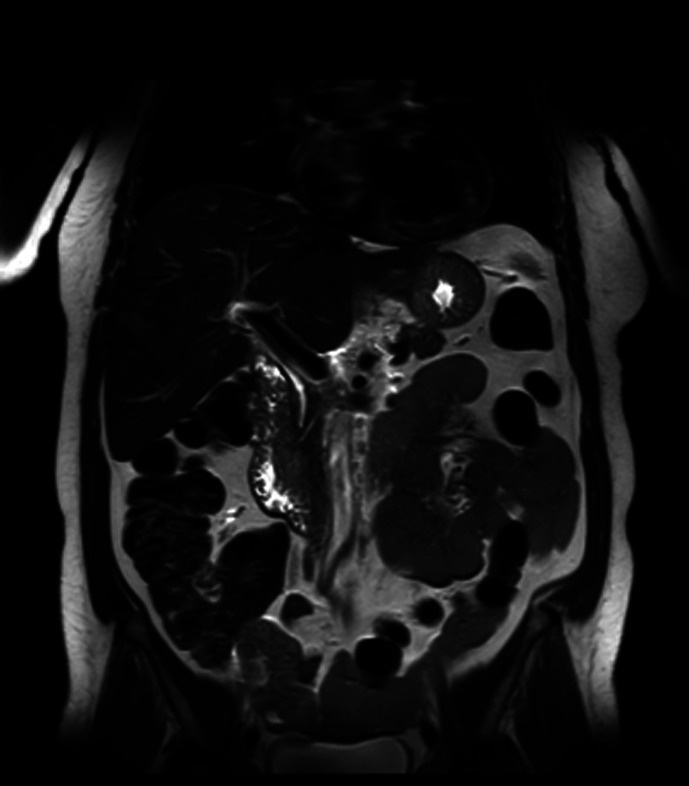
Coronal T2-weighted MR image showing a broad-based lesion at the ampulla. MR, magnetic resonance.
